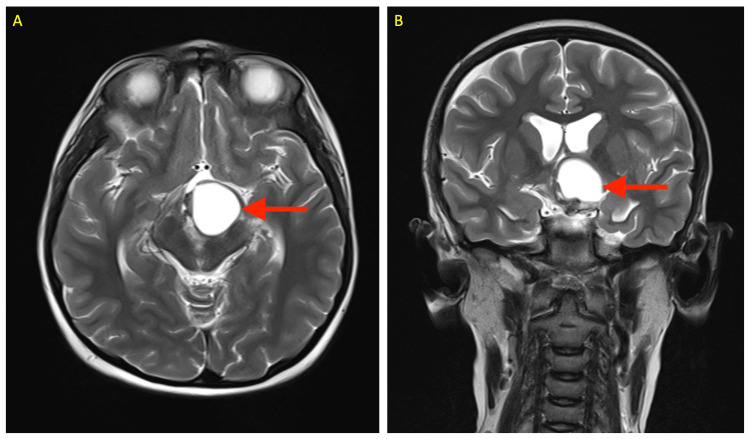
T2 weighted MRI scans showing residual craniopharyngioma in the sellar-suprasellar region of brain (shown by red arrows): (A) Axial view, (B) Coronal view
